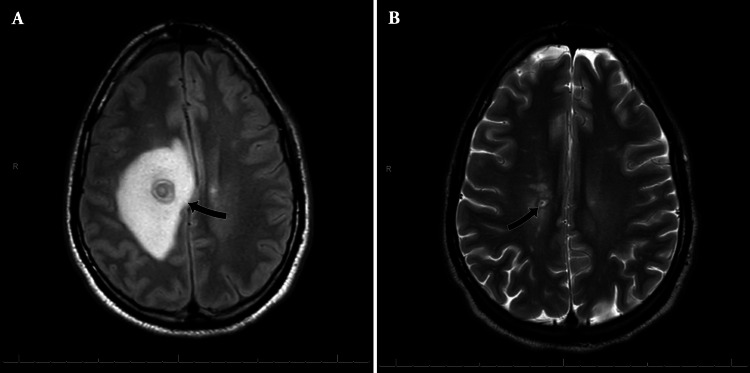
A) MRI with intravenous gadolinium showed a lesion with significant vasogenic edema and midline shift; B) Post-craniotomy MRI showing residual lesion.
